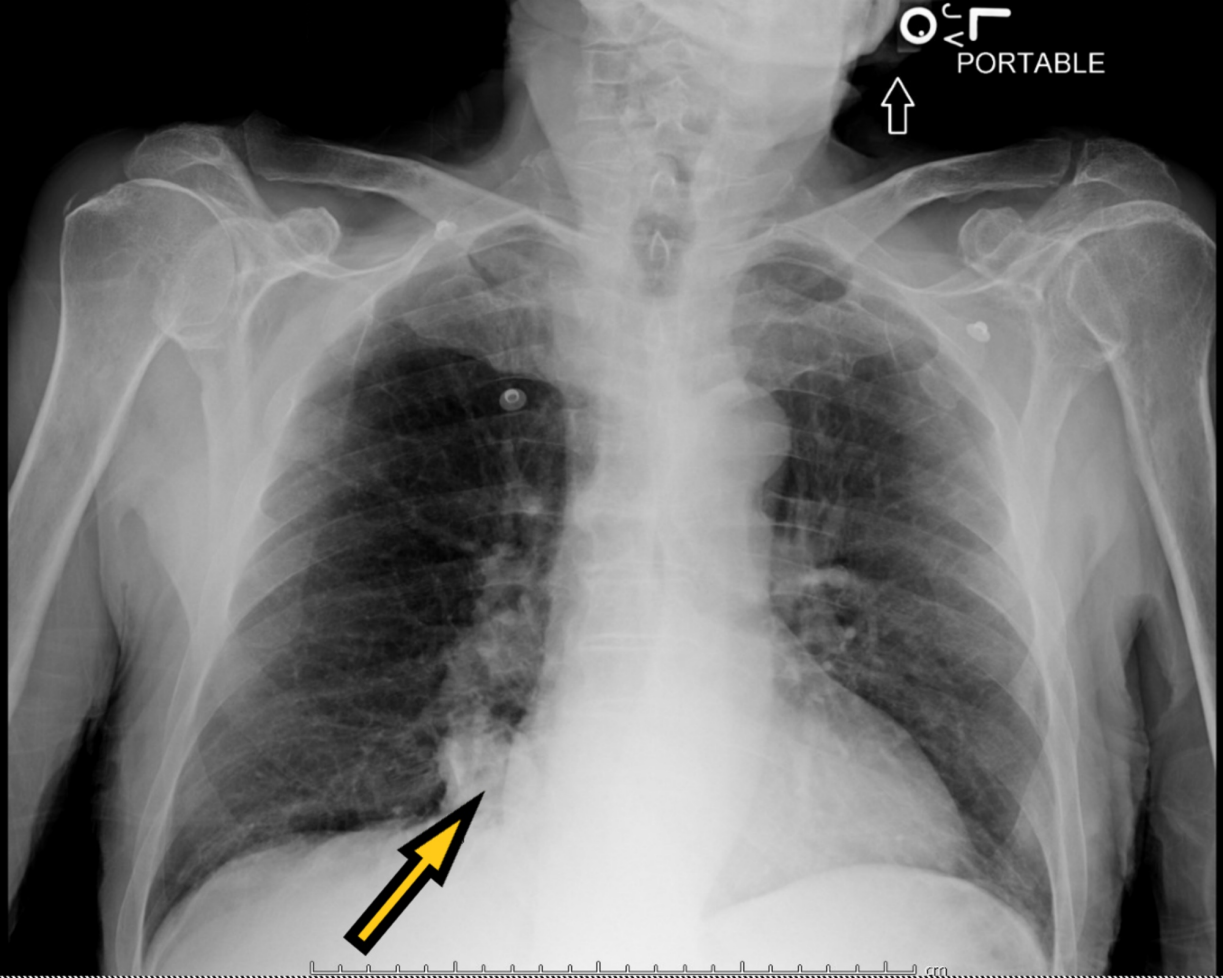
Chest X-ray showing focal consolidation in the medial right lung base.
